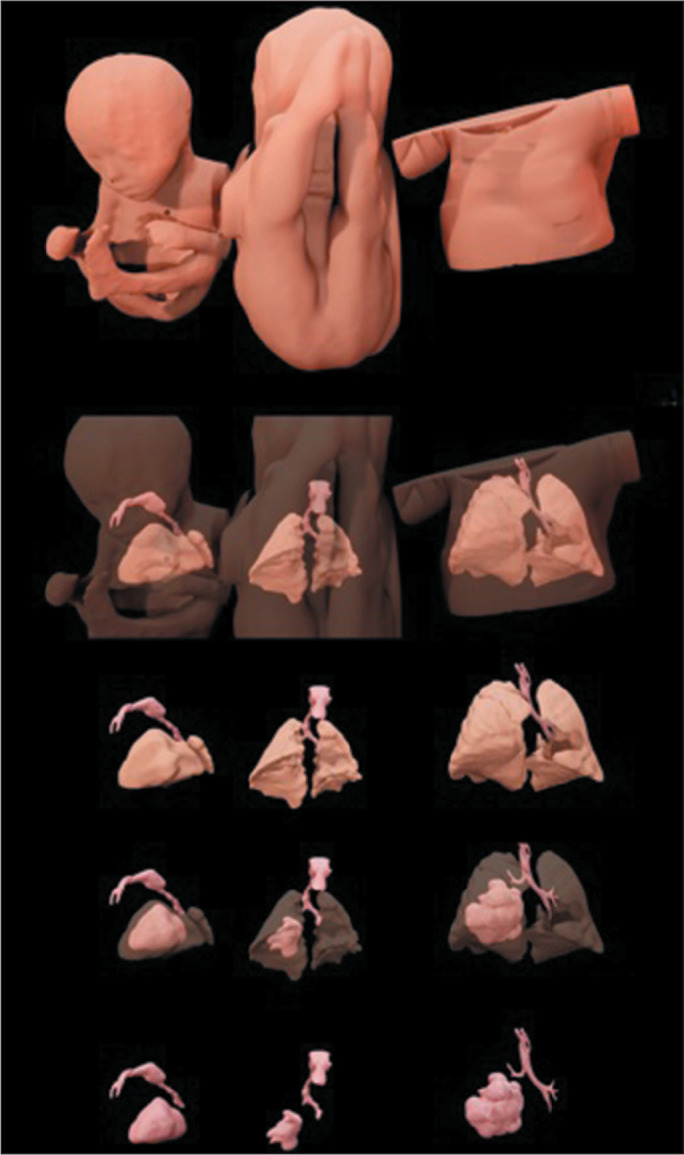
3D reconstructions from fetal MRI scans acquired at 25 weeks of gestation
